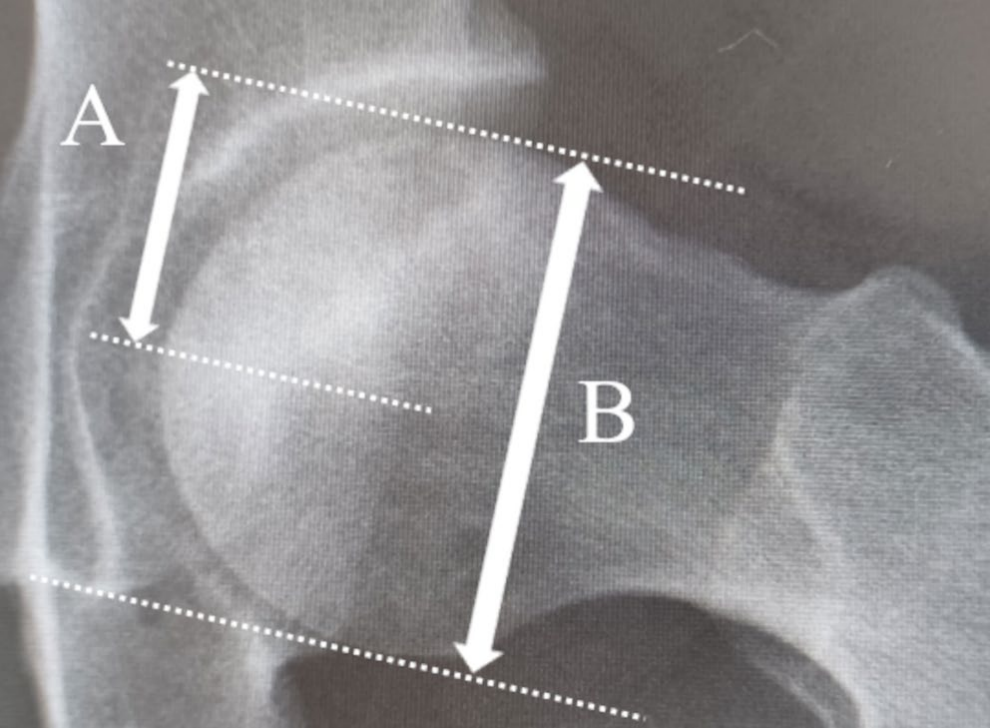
The extent of the anteroposterior (AP) necrotic lesions was measured as the ratio of the longest AP length of the necrotic lesion (A) to the largest AP diameter of the femoral head (B) on lateral radiograph
